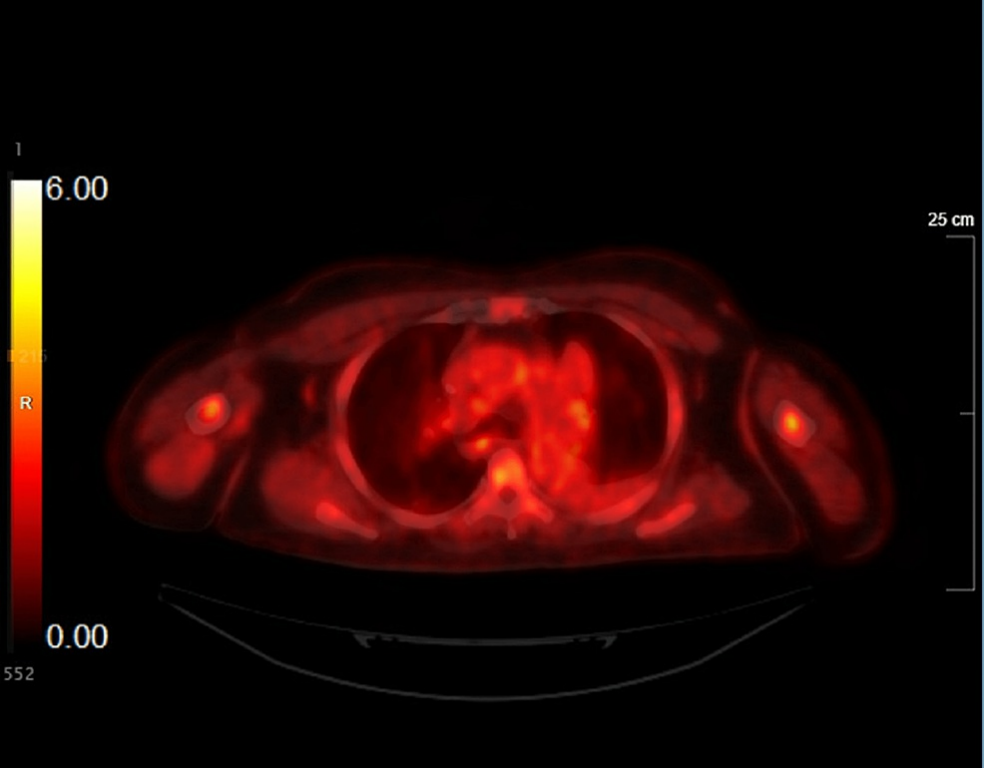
FDG-PET scan showing improving FDG avidity in the subcarinal LN (January 2023) FDG, fluorodeoxyglucose, PET, positron emission tomography, LN, lymph node
